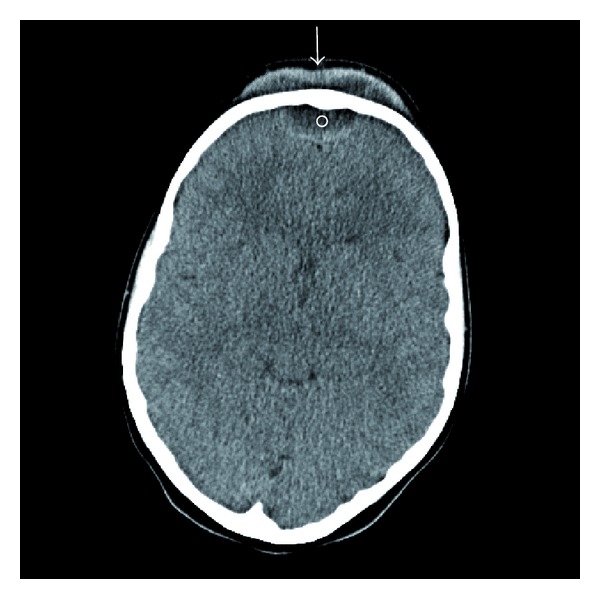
Axial CT of the brain demonstrating subperiosteal (arrow) and epidural abscesses (circle).
